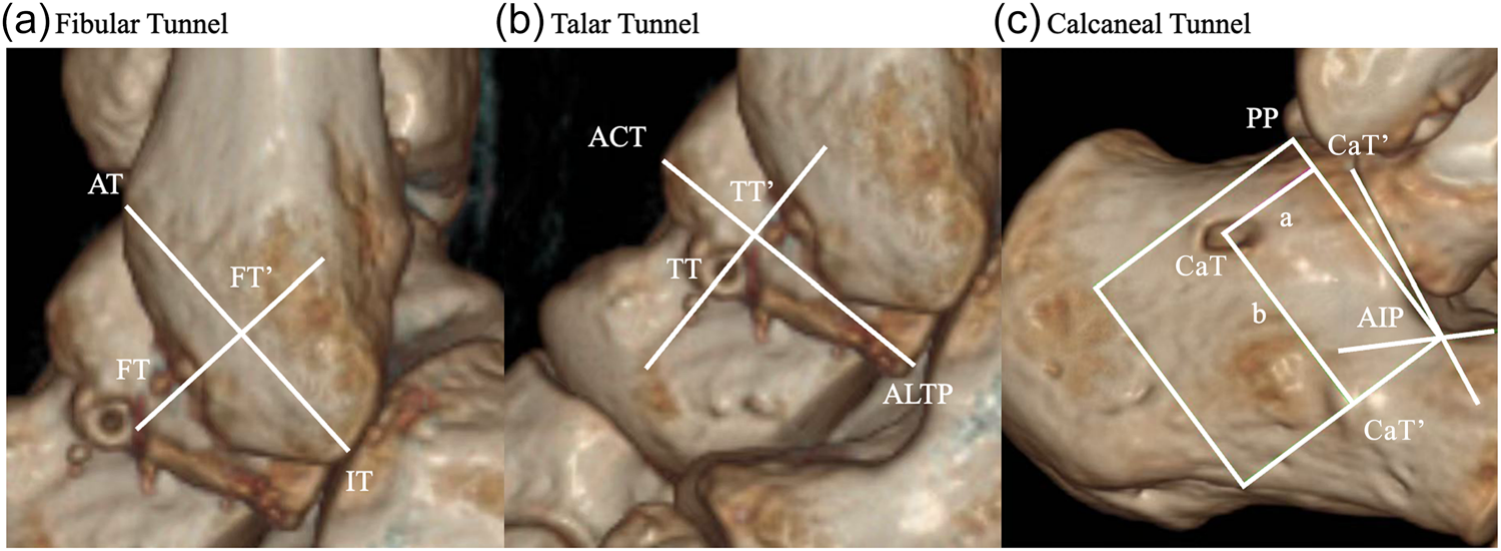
3D‐CT measurement methods of the (a) fibular, (b) talar and (c) calcaneal tunnel position. 3D‐CT, three‐dimensional computed tomography; ACT, anterolateral corner of the trochlea; AIP, anteroinferior point; ALTP, apex of the lateral talar process; AT, anterior tubercle of the fibula; CaT, calcaneal tunnel; CaT′, the projection point of the entrance of the calcaneal tunnel; FT, fibular tunnel; FT′, the projection point of the entrance of the fibular tunnel; IT, inferior tip of the fibula; PP, posterior point of the posterior subtalar joint; TT, talar tunnel; TT′, projection point of the entrance of the talar tunnel.
